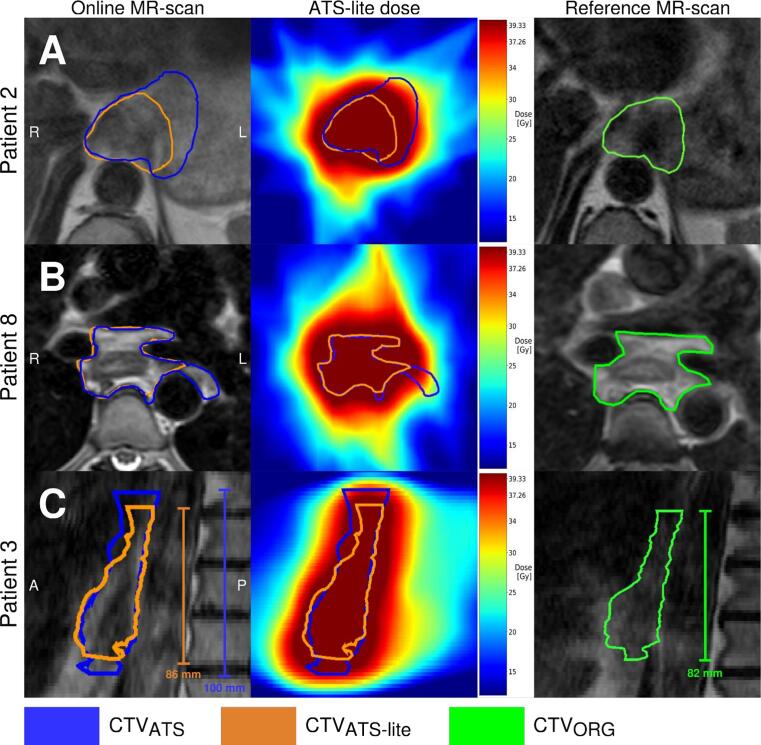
Examples of fractions with inadequate coverage of the clinical target volume (CTV) defined in the clinical ATS procedure (CTV_ATS_) for patients 2 (top), 8 (middle), and 3 (bottom). For each fraction, the unadjusted propagated CTV on which the treatment plan was optimized (CTV_ATS-lite_, orange) and the CTV_ATS_ (blue) are depicted on the online MRI (left) and the estimated delivered dose distribution (middle). The reference MRI (right) shows the reference CTV used for deformable contour propagation (CTV_ORG_, green). Notable differences between the CTV_ORG_ and the CTV_ATS_ can be observed for all three fractions.
